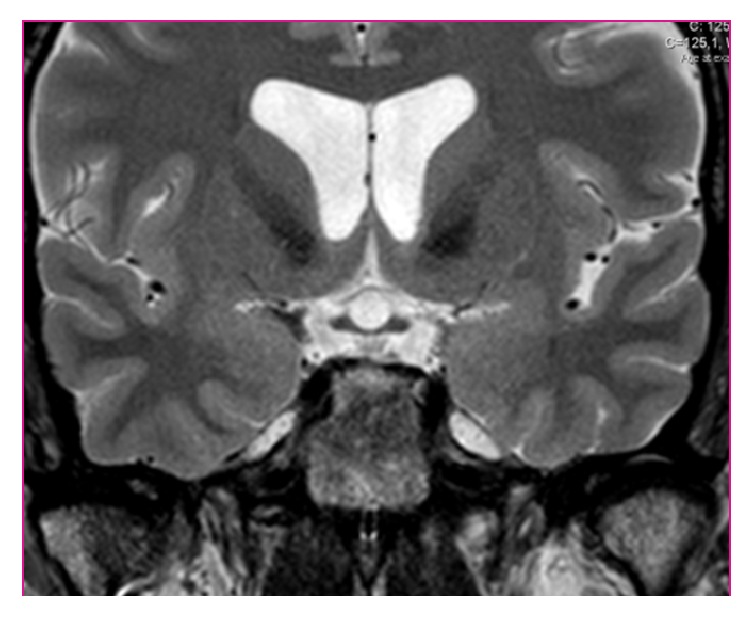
MRI of* sella turcica* at 10-month follow-up (May 2015): normal pituitary size with discrete heterogenous enhancement.
